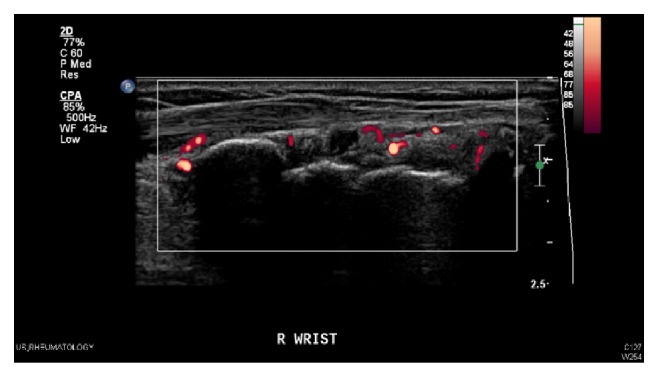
Wrist joint with Power Doppler synovitis in patient with RA.
